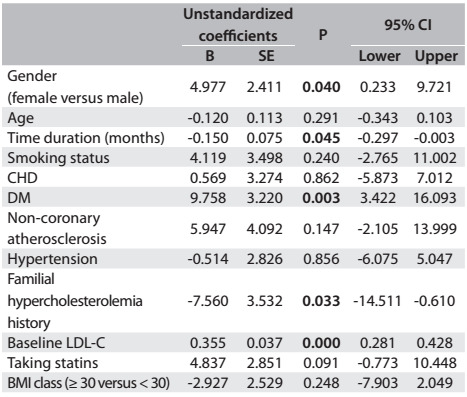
Magnetic resonance imaging of spinal cord, two months after admission, showing: hyperintense image with T2 weighting (A); and enhancement after gadolinium-diethylenetriamine pentaacetic acid (Gd-DTPA) administration (B).
